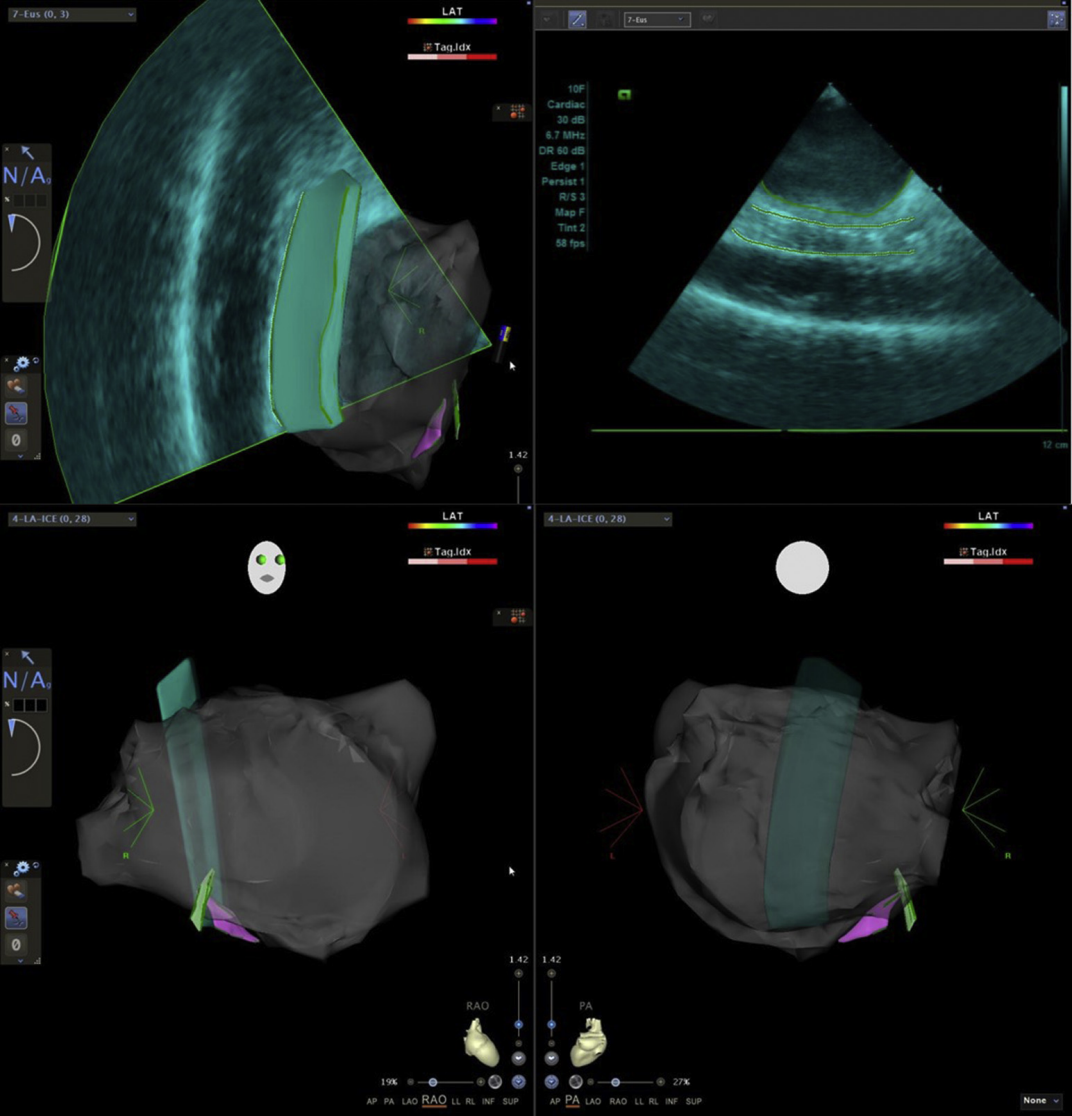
A 3D reconstruction of the left atrium, pulmonary veins, and the esophagus with SoundStar catheter (3D diagnostic ultrasound catheter, 10F, 90 cm; Biosense Webster, Inc, Diamond, Bar, CA) and CartoSound mapping system. The esophagus temperature probe is also visible.
